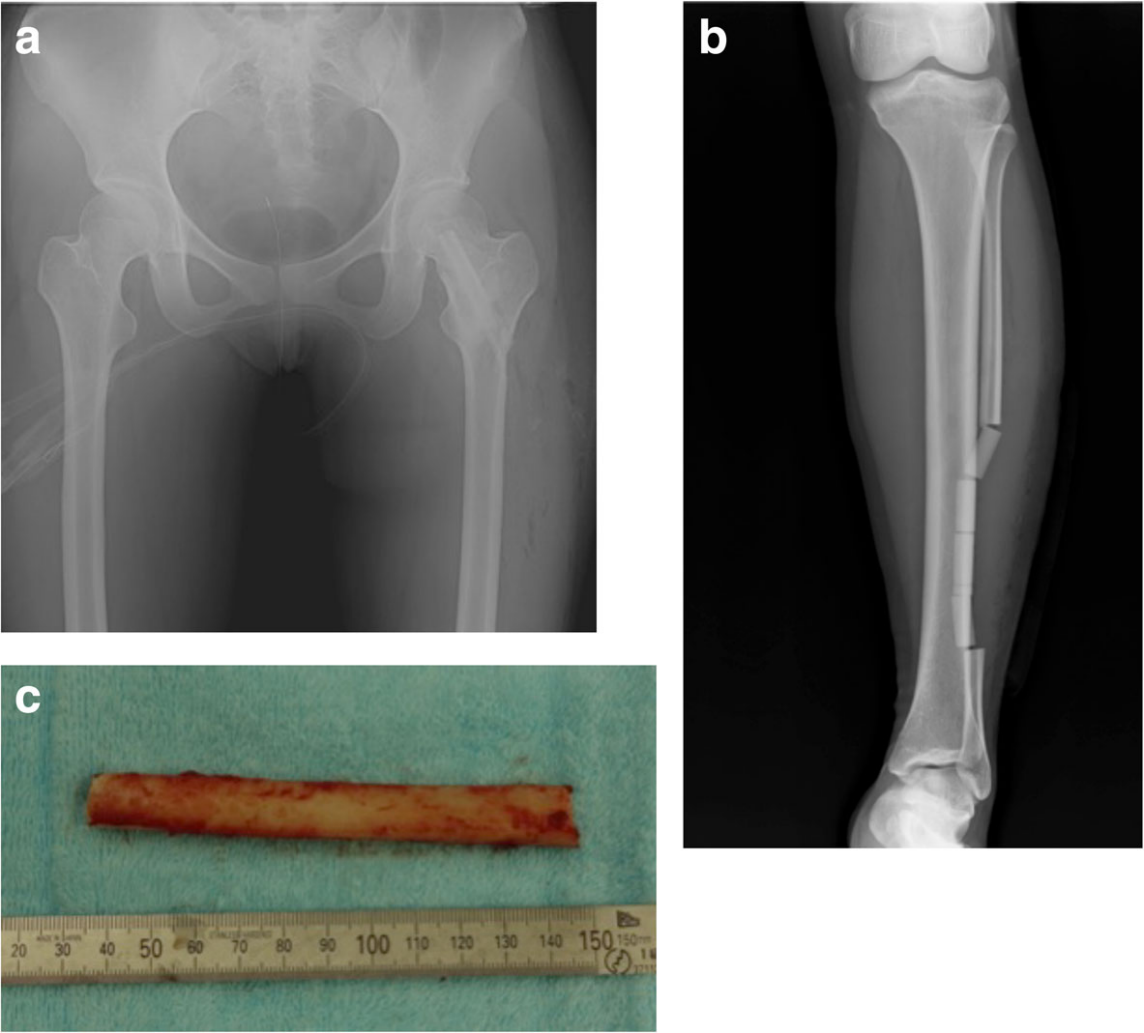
Postoperative radiograph of the neck of femur with left autologous fibula graft strut inserted. a Postoperative radiograph of the neck of femur. b The defect in the fibula was filled with β-tricalcium phosphate (Superpore®; HOYA Technosurgical Inc., Japan). c left autologous fibula graft strut inserted
